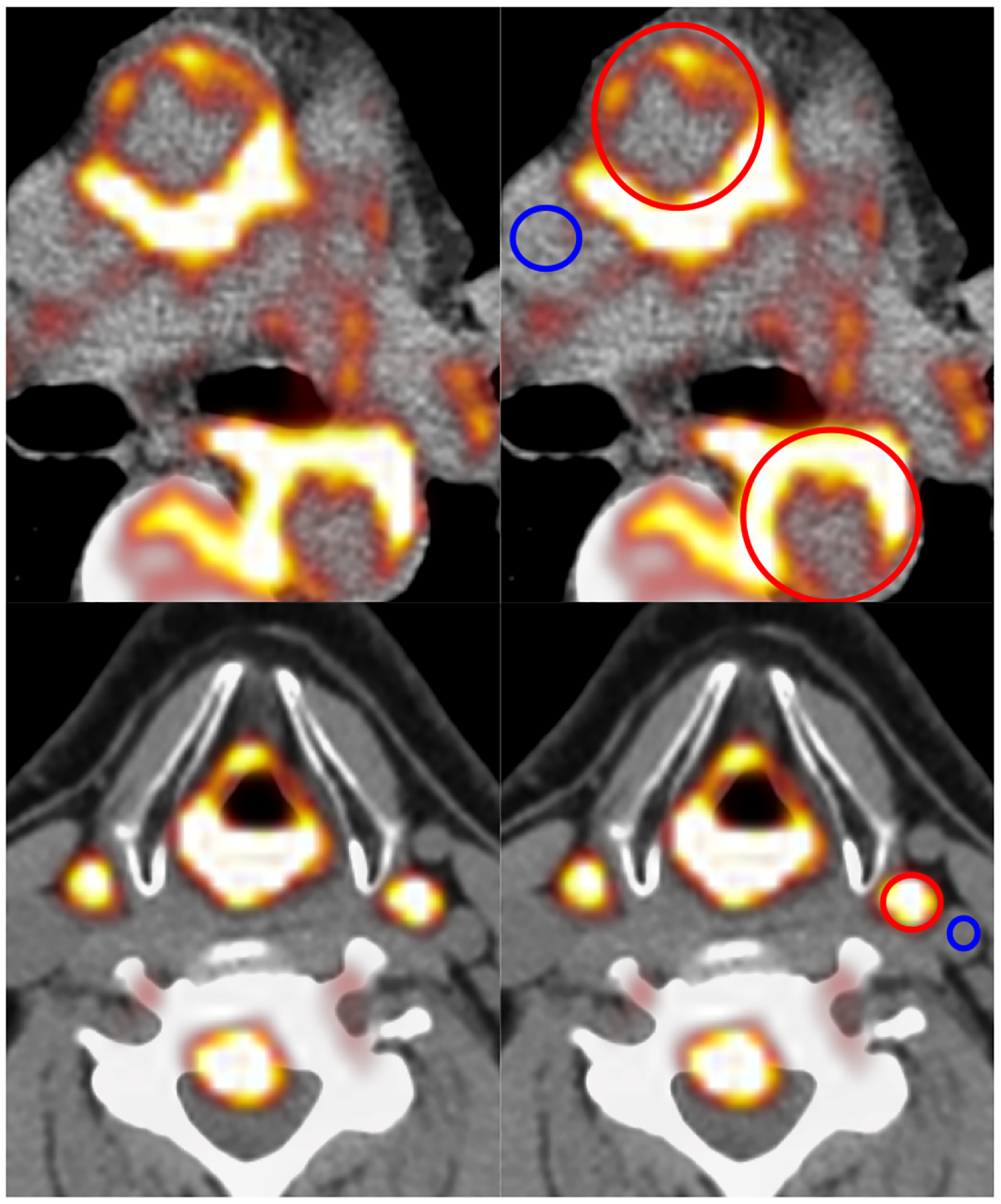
Calculation of arterial tissue-to-blood ratio (TBR) as a measure of arterial inflammation. Calculation of TBR: ratio of FDG tracer concentration in the artery divided by FDG concentration in the venous blood pool (superior vena cava for aorta, internal jugular for carotid).
